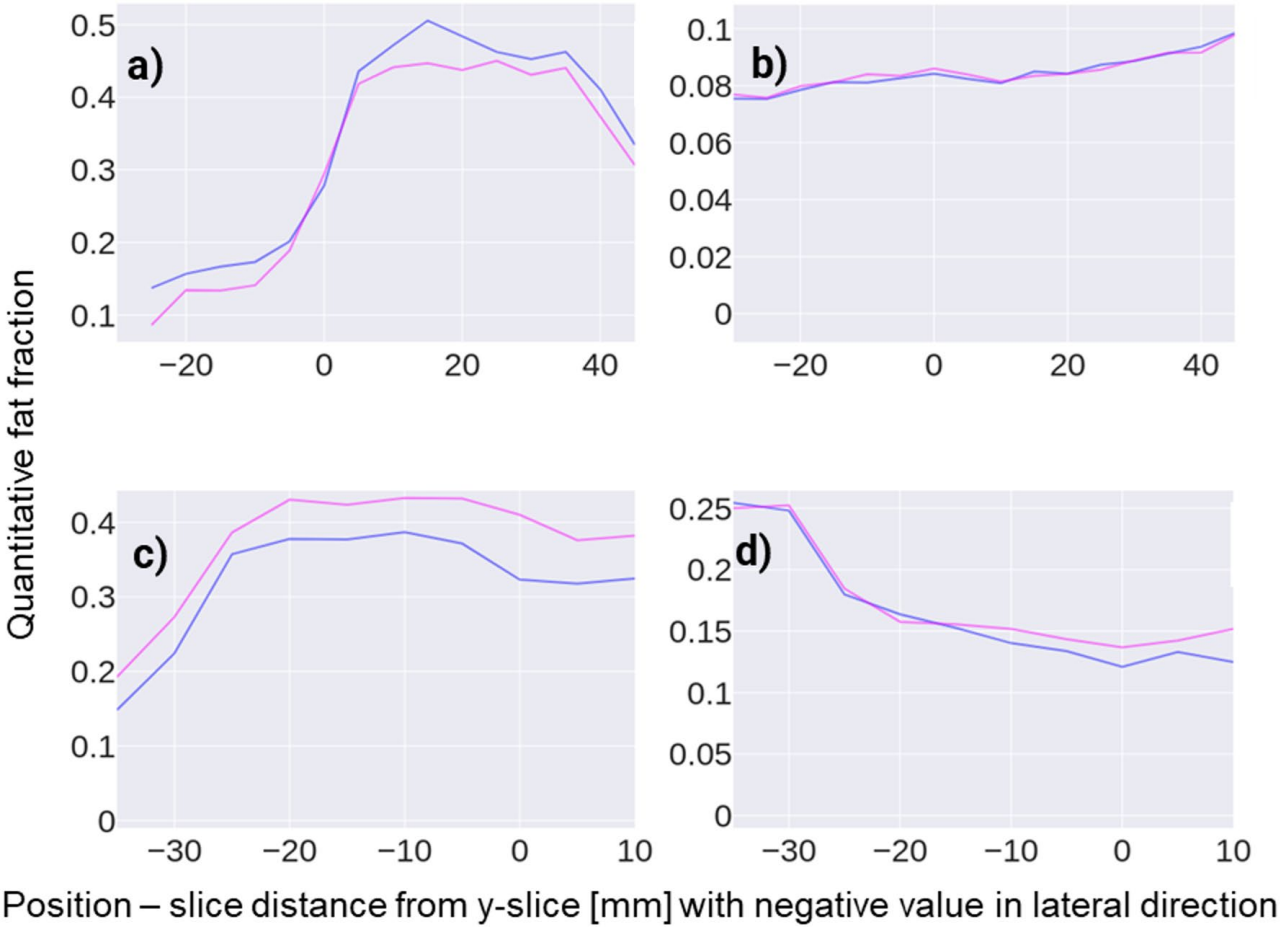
Predicted (magenta) and ground-truth (blue) quantitative fat fraction distribution of individual muscles: subscapularis muscle in coronal MRI with increased fat fraction medial to the Y-slice a) and subscapularis muscle in coronal MRI with constant fat fraction; b) supraspinatus muscle in sagittal MRI with decreased c) and increased fat fraction in the lateral segment.
